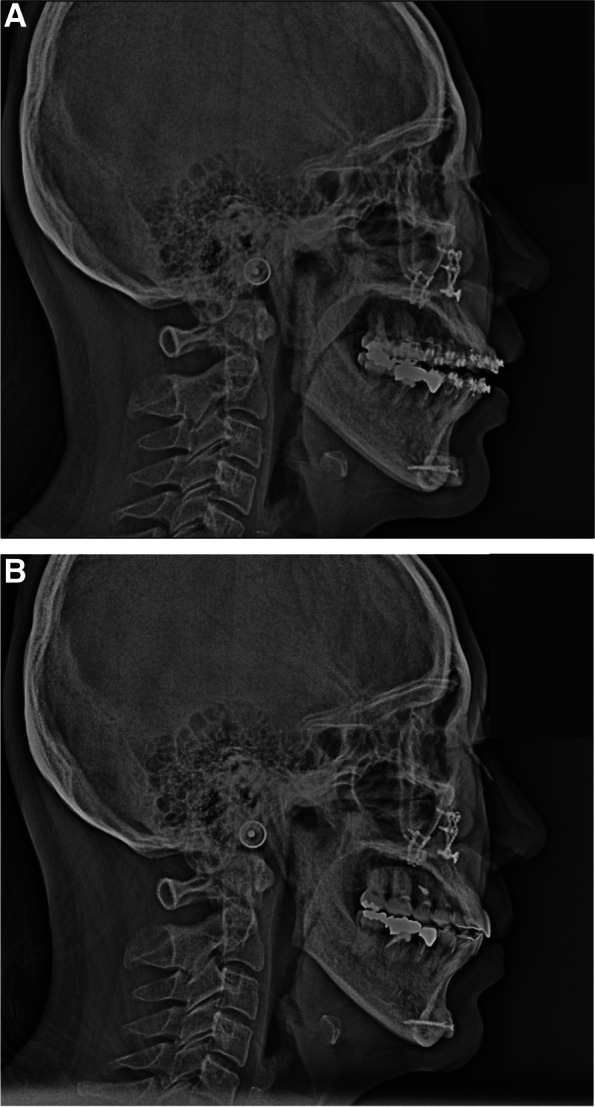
Postoperative cephalometric radiography. A Lateral cephalometric radiography immediately after orthognathic surgery. Maxilla was done by Le Fort I osteotomy and positioned postero-superiorly. The mandible was repositioned posteriorly through vertical ramus osteotomy. The inferior part of the proximal segment of the mandible was fixed to the mentum, and the surgery was carried out as planned. B Lateral cephalometric radiograph 1.5 years after surgery, grafted proximal segment inferior part bone of the mandible was slightly absorbed and bone fused to the mentum of the mandible
